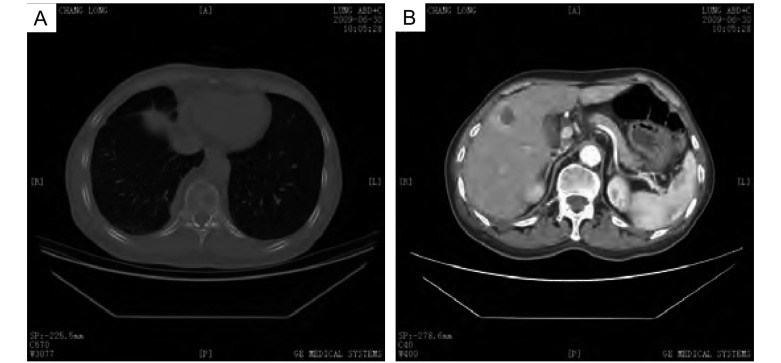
转移癌的CT显像。A：骨转移癌，B：肝转移癌 CT image of metastases. A: bone metastasis; B: hepatic metastasis
